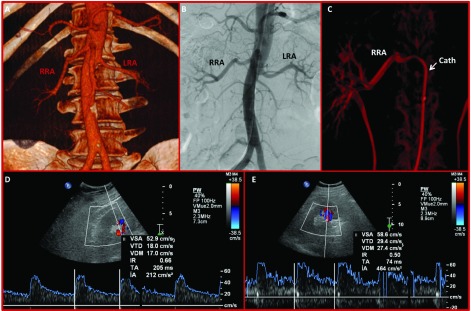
Multi-Modality Imaging For Renal Denervation A) 3D-angio-computerized tomography (CT) showing anatomic characteristics of the right and left renal arteries (RRA, LRA, respectively); B) Angiographic appearance of the renal arteries after contrast injection; C) 3D-angiography reconstruction of the RRA showing a curvature of the mid-RRA; D) RRA and E. LRA Doppler velocity showing normal waves
